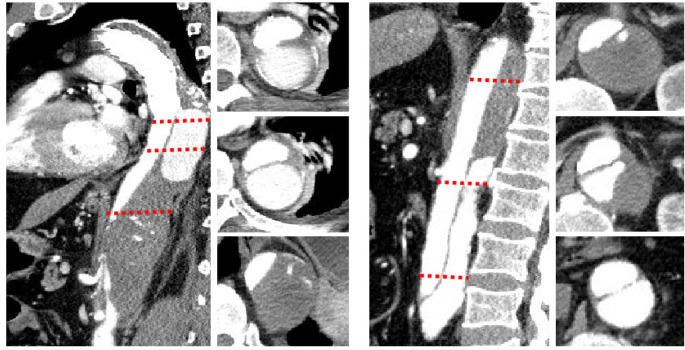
Computed tomography angiography image after onset of paraplegia in our
